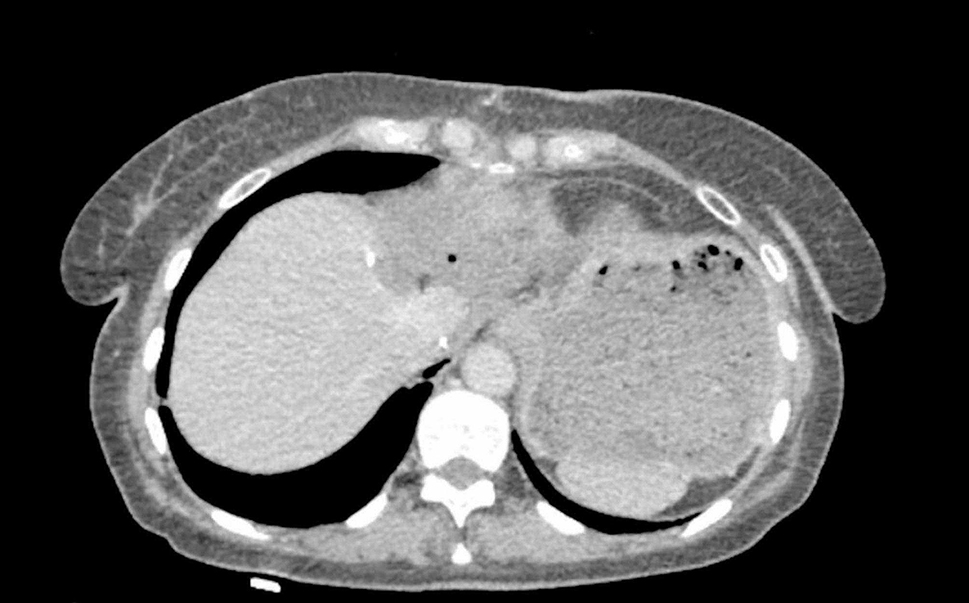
CT scan after 3 years. There is no data for recurrent
